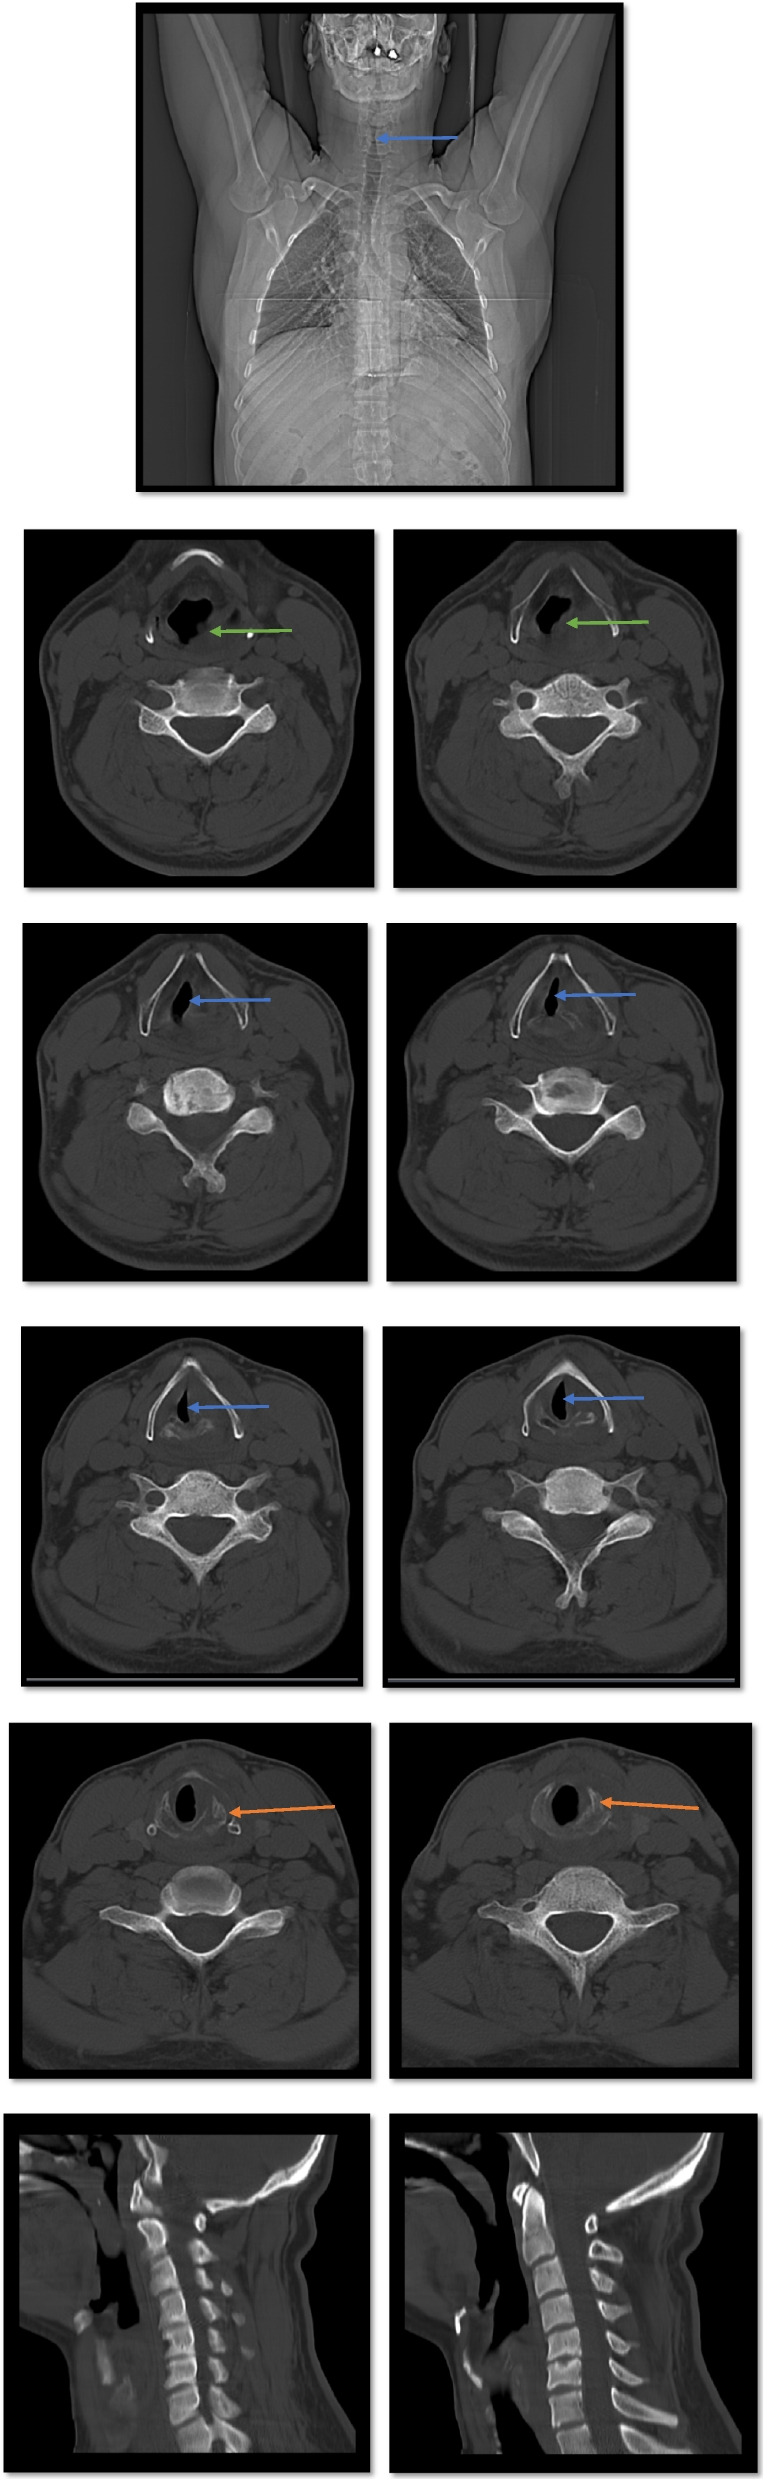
Spiral neck CT scan with IV contrast showing cricoid cartilage fracture and narrowing of the airway (axial and sagittal views). Green arrows show mucosal thickening, inflammation, and edema extended to the glottis, blue arrows show narrowing of the airway, and orange arrows show the cricoid fracture
